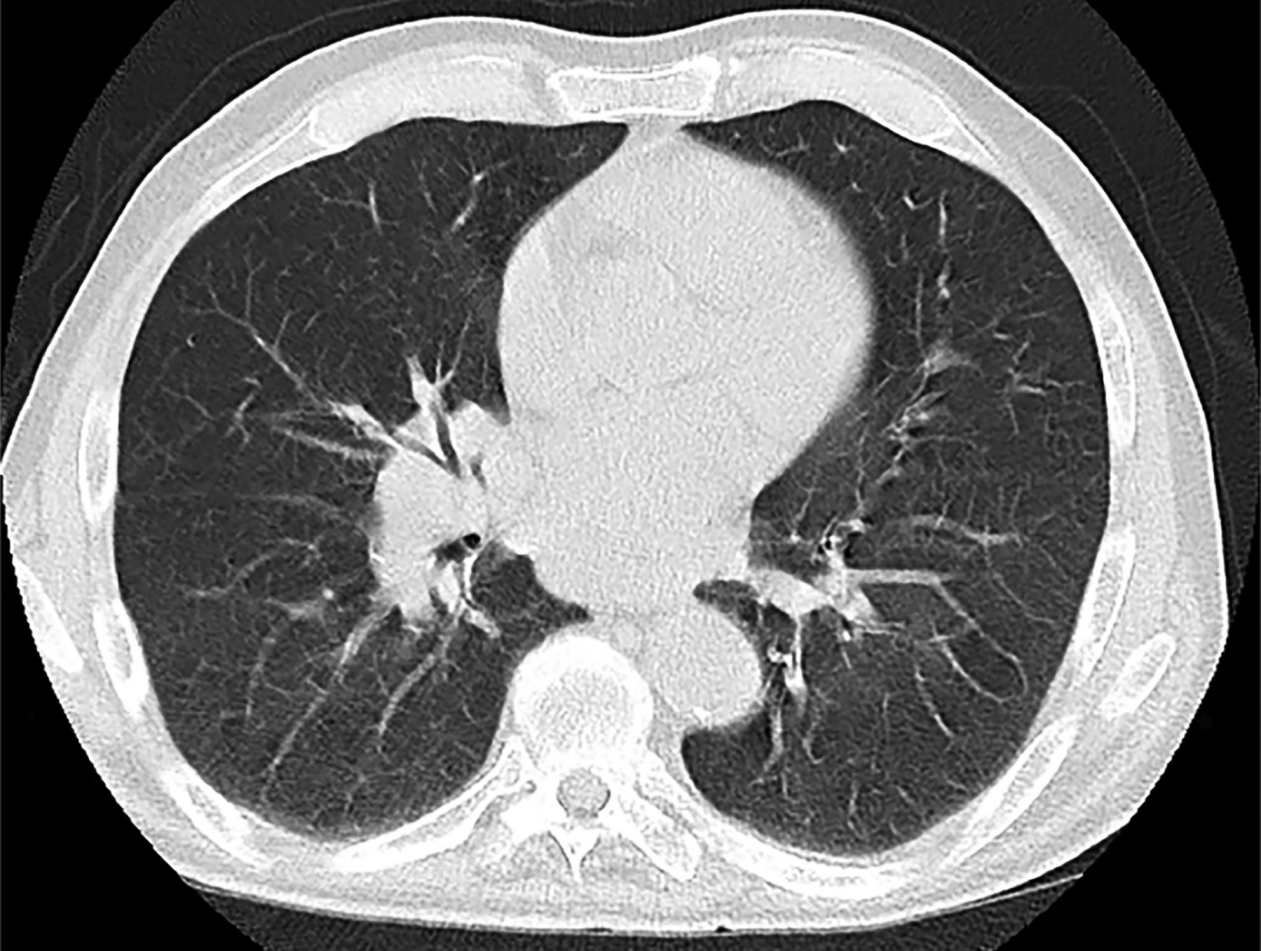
High‐resolution CT (HRCT) of the chest showing hilar lymphadenopathy.
